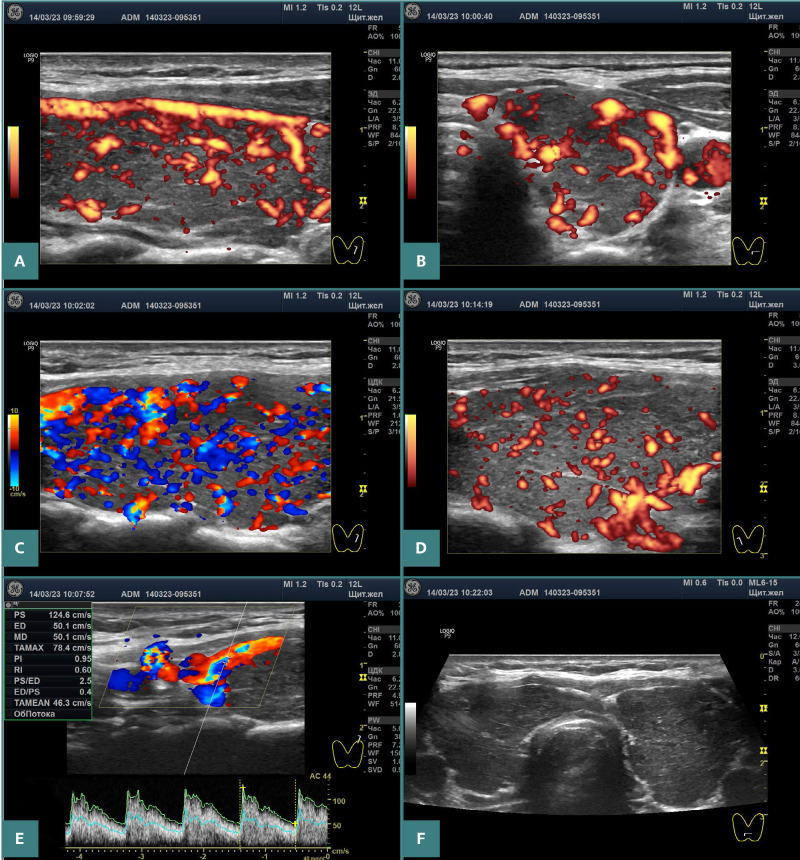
Ultrasound images of the thyroid of patient 1. A,B, Significant increase in blood flow intensity in the left lobe in power Doppler mode at optimal PRF. C, ‘Thyroid inferno’ sign in the left lobe in color Doppler mode at low PRF. D, Significant increase in blood flow intensity in the right lobe in power Doppler mode at optimal PRF. E, PSV in the first branch of the STAs on the left: 124.6 cm/s. F, Both lobes in grayscale mode.
